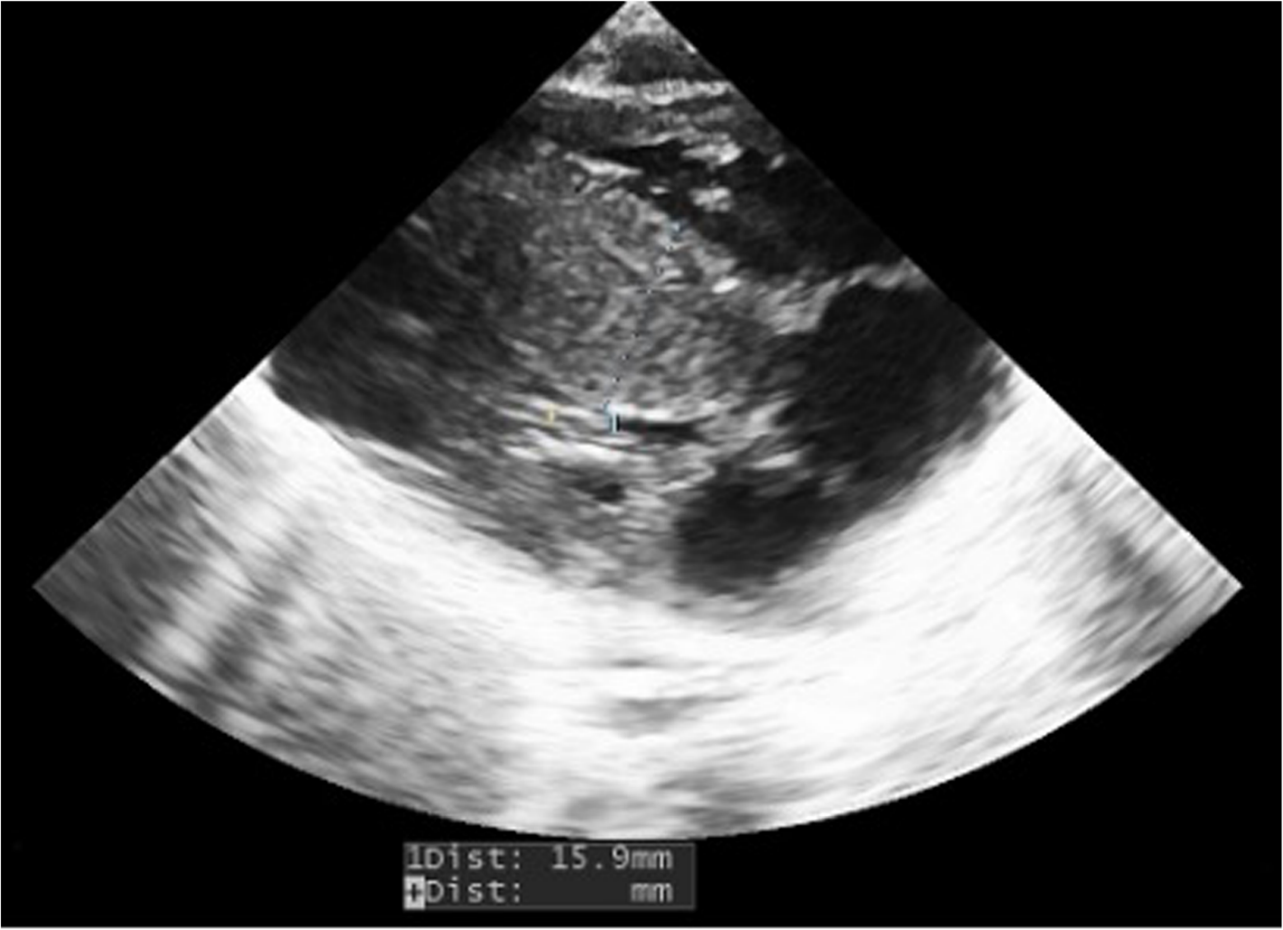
Echocardiogram performed after birth showed severe Left Ventricular (LV) hypertrophy and interventricular septal hypertrophy (IVSd of 15.9 mm, z-score 6.81) with a significant reduction in the ventricular cavity
